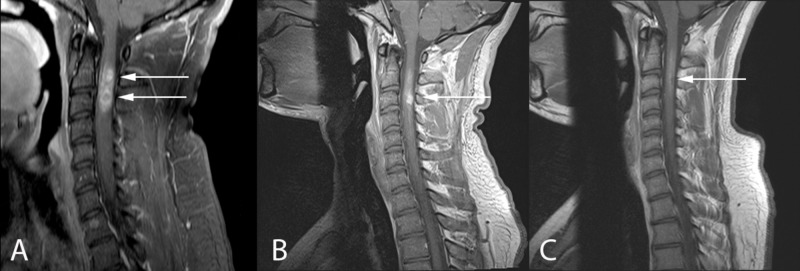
Sagittal T1-weighted MRI with gadolinium at patient presentation (A), three-week follow-up (B), and nine-week follow-up (C) depicting almost complete spontaneous resolution of the cervical intramedullary spinal cord lesion (arrows)
